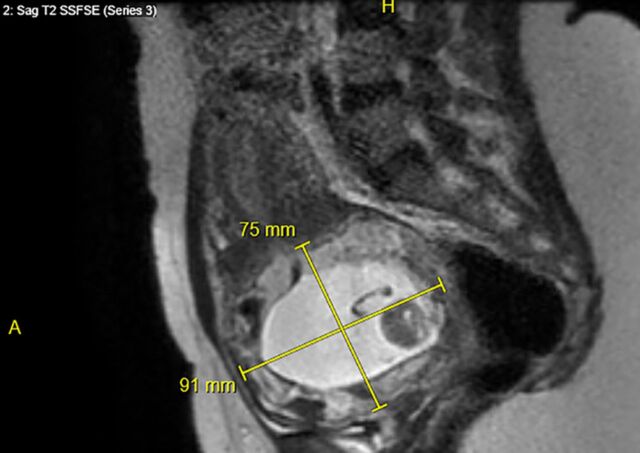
Sagittal pelvic magnetic resonance imaging demonstrating the cervical ectopic pregnancy with measurement of the gestational tissue mass (91 mm × 75 mm).
